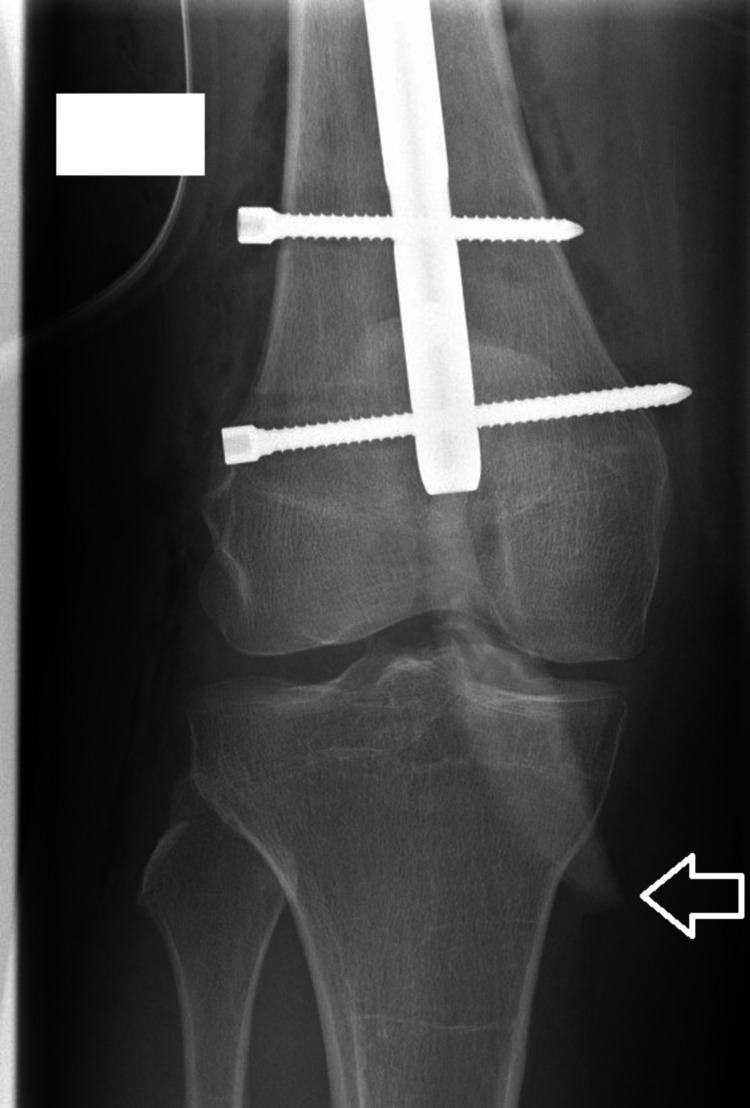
White arrow marks the intraarticular bone fragment on an anteroposterior postoperative X-ray
